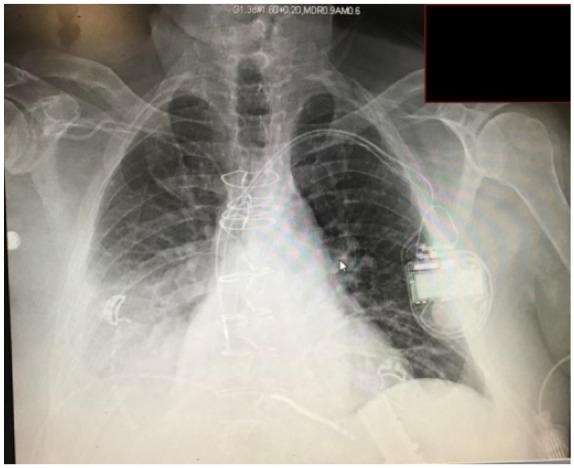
Chest X-ray shows unilateral opacification in right lower lobe.
